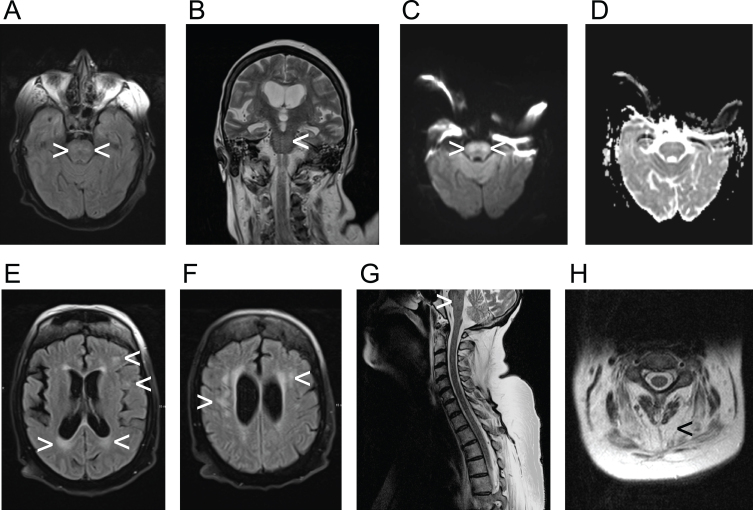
Demonstration of radiological findings in the patient, diagnosed with myotonic dystrophy type 1, utilizing various acquisition techniques. A) fluid-attenuated inversion recovery (FLAIR), axial view; B) coronal T2-weigthed image; C) axial view, diffusion-weighted magnetic resonance imaging (DWI); D) axial view, apparent diffusion coeffizient (ADC); E) and F) fluid-attenuated inversion recovery (FLAIR), axial views; G) T2-weigthed image, saggital view; H) T2-weigthed image, axial view with highlighted sign of muscular atrophy. Arrowheads are implemented to highlight pathologies exemplary. The participant provided an informed consent to publish health data including imaging data.
